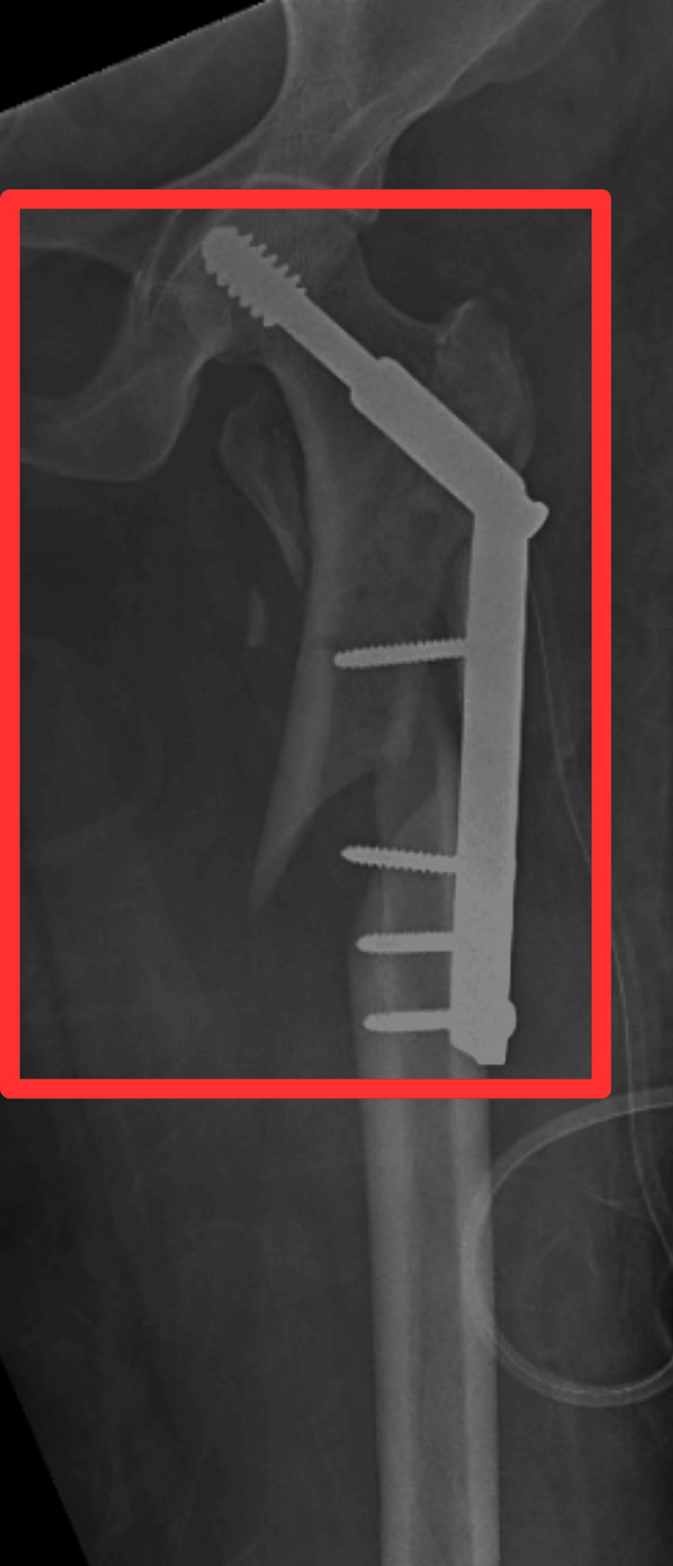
X-ray showing the surgical correction of the subtrochanteric fracture of the left side The red rectangle shows the open reduction and internal fixation done with the help of screws and plates
